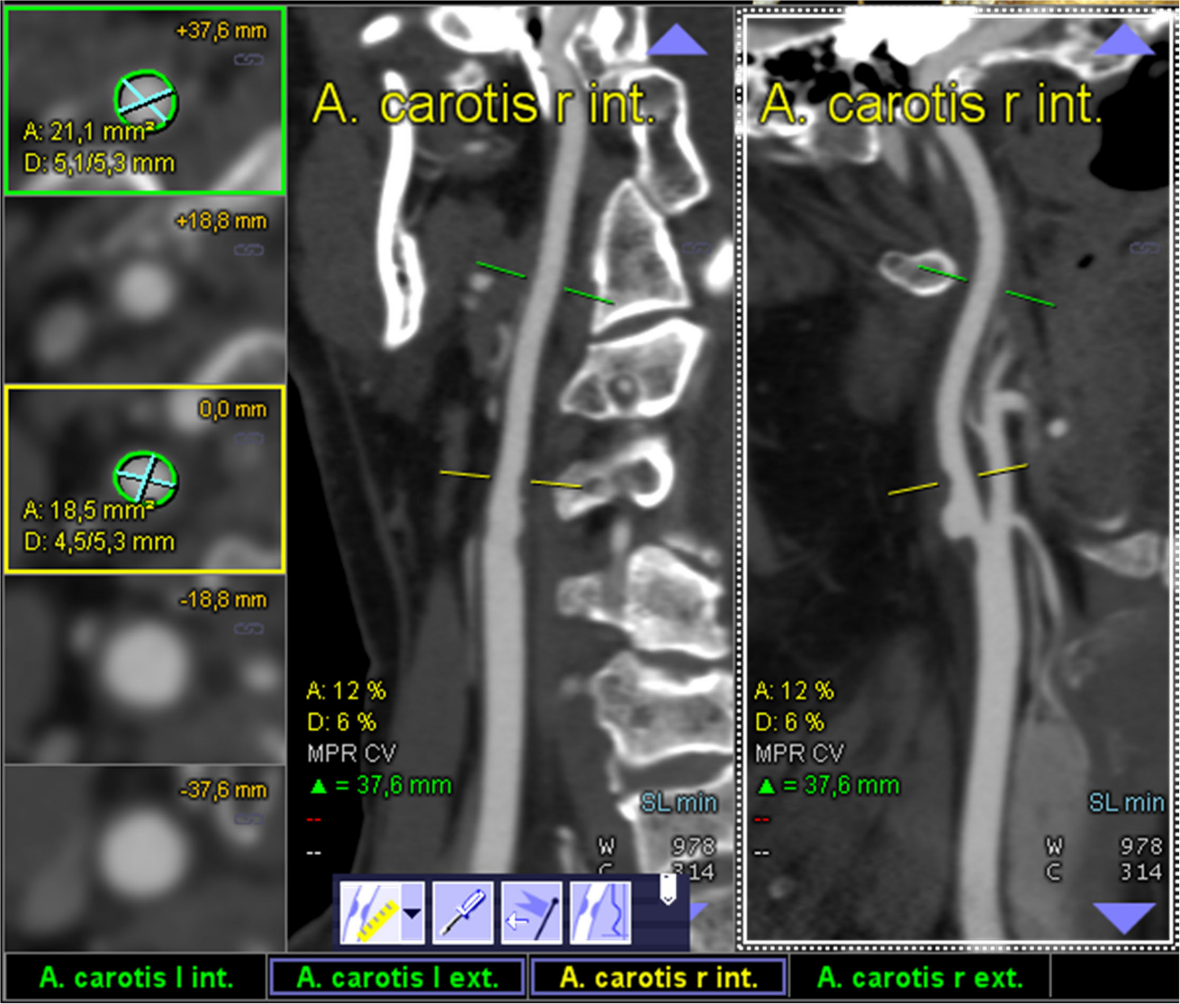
Display of an optimal semiautomated perpendicular stenosis area minimal caliber computation as provided by the “syngo.via” image analysis software: one of the ICAs is automatically detected and the sufficiently contrasted CTA data are displayed as multiplanar reformatted coronal and sagittal view (middle and left column; centerline faded). The segmentally determined luminal area and the underlying non-perpendicular maximum calibers are displayed in the left column (digital caliper).
